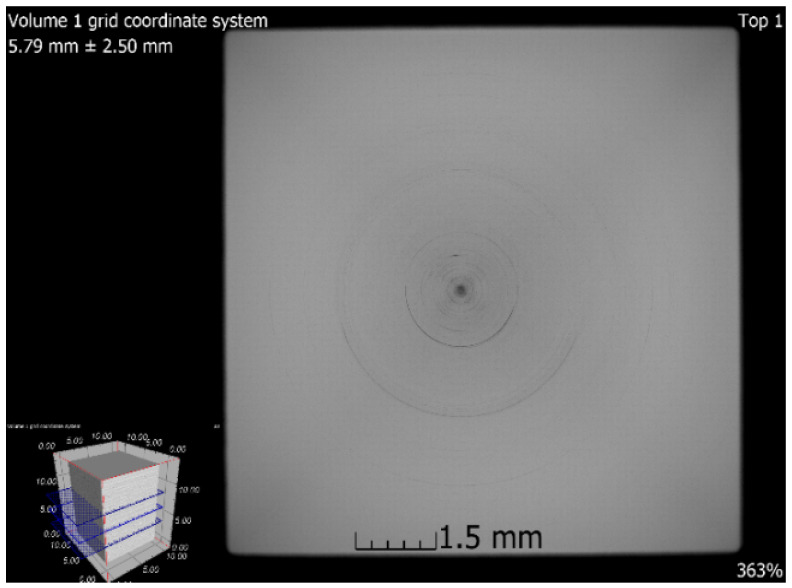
Tomographic CT sections of sample 5 after SLM + HIP_2 (XY plane).
